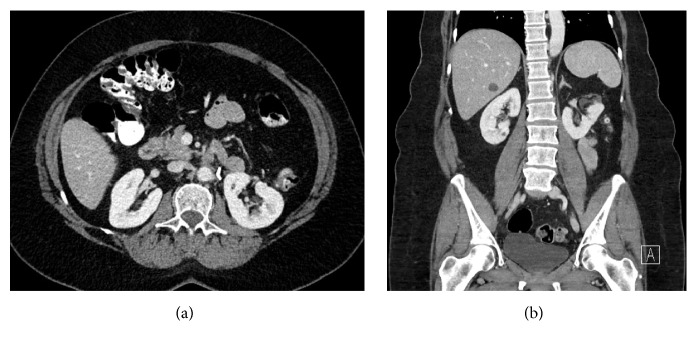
Axial (a) and coronal (b) views of abdominal CT showing recovered left kidney, ten months after partial nephrectomy for removal of a giant AML.
